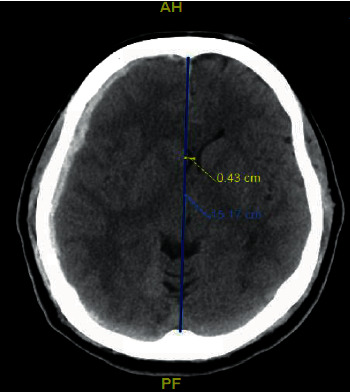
Noncontrast axial CT head showing the right subdural hematoma with right to left midline shift of 4 mm.
